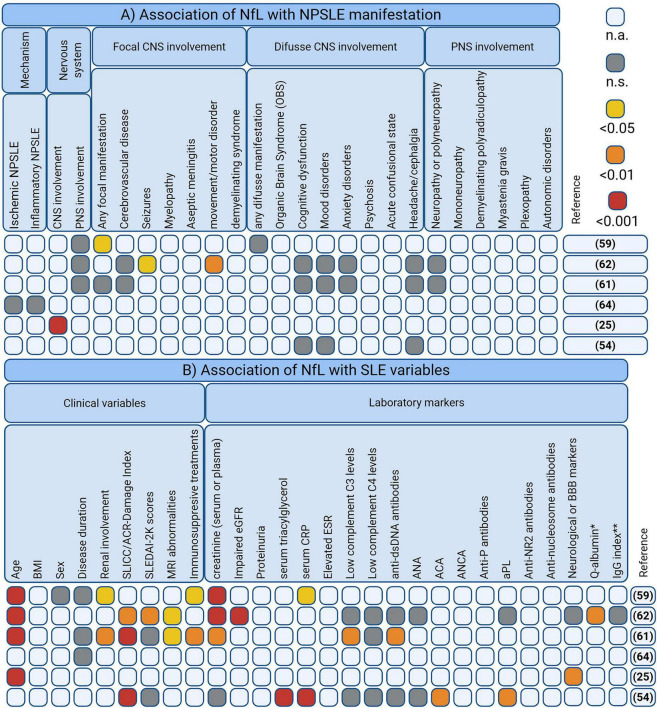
Comparative map of clinical variables related to serum NfL levels. (A) Associations of NfL with NPSLE manifestations. (B) Associations of NfL with demographic and clinical variables in SLE. This figure provides a comparative overview of how different studies, using diverse statistical approaches, have examined the relationship between serum NfL and clinical variables. Readers can interpret the grid by following the color coding, which indicates statistical significance: Red, *p* < 0.001; Orange, *p* < 0.01; Yellow, *p* < 0.05; Gray, not significant; Blue, not assessed. In this way, the figure highlights both consistently reported associations and underexplored aspects. Further details of each association are described in the main text. Neurofilament light; NPSLE, neuropsychiatric systemic lupus erythematosus; CNS, central nervous system; PNS, peripheral nervous system; BMI, body mass index; SLICC/;ACR, systemic lupus international collaborating clinics/american college of rheumatology; SLEDAI-2K, systemic lupus erythematosus disease activity index 2000; MRI, magnetic resonance imaging; eGFR, estimated glomerular filtration rate; CRP, C-reactive protein; ESR, erythrocyte sedimentation rate; anti-dsDNA antibodies, anti-double-stranded deoxyribonucleic acid antibodies; ANA, antinuclear antibodies; ACA anticentromere antibodies; ANCA, anti-neutrophil cytoplasmic antibodies; Anti-P, anti-ribosomal P; aPL, antiphospholipid antibodies; NfL, neurofilament light chain. Q-albumin, quotient albumin*, is the ratio between CSF/Serum albumin, higher Q-albumin indicates BBB disruption. IgG index [(CSF/S IgG ratio)/(CSF/S albumin ratio)], higher IgG index indicates IgG CNS production. Created with BioRender.com.
